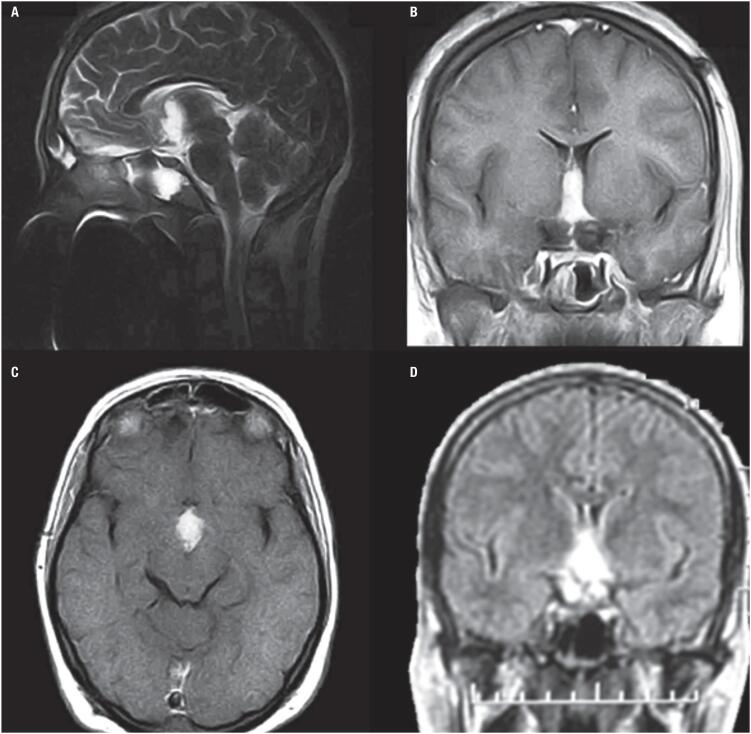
Patient 1. Postoperative and pre-radiotherapy magnetic resonance imaging findings. (A) The sagittal T2 weighted image showed remaining tumor at the third ventricle but without no significant mass effect. (B) The coronal T1 gadolinium weighted image showed the tumor inside the third ventricle but with ventricular symmetry. (C) The axial T1 gadolinium weighted image showed remnant tumor in the interpeduncular cistern. (D) The FLAIR sequence showed spontaneous brightness of the chordoid glioma.
